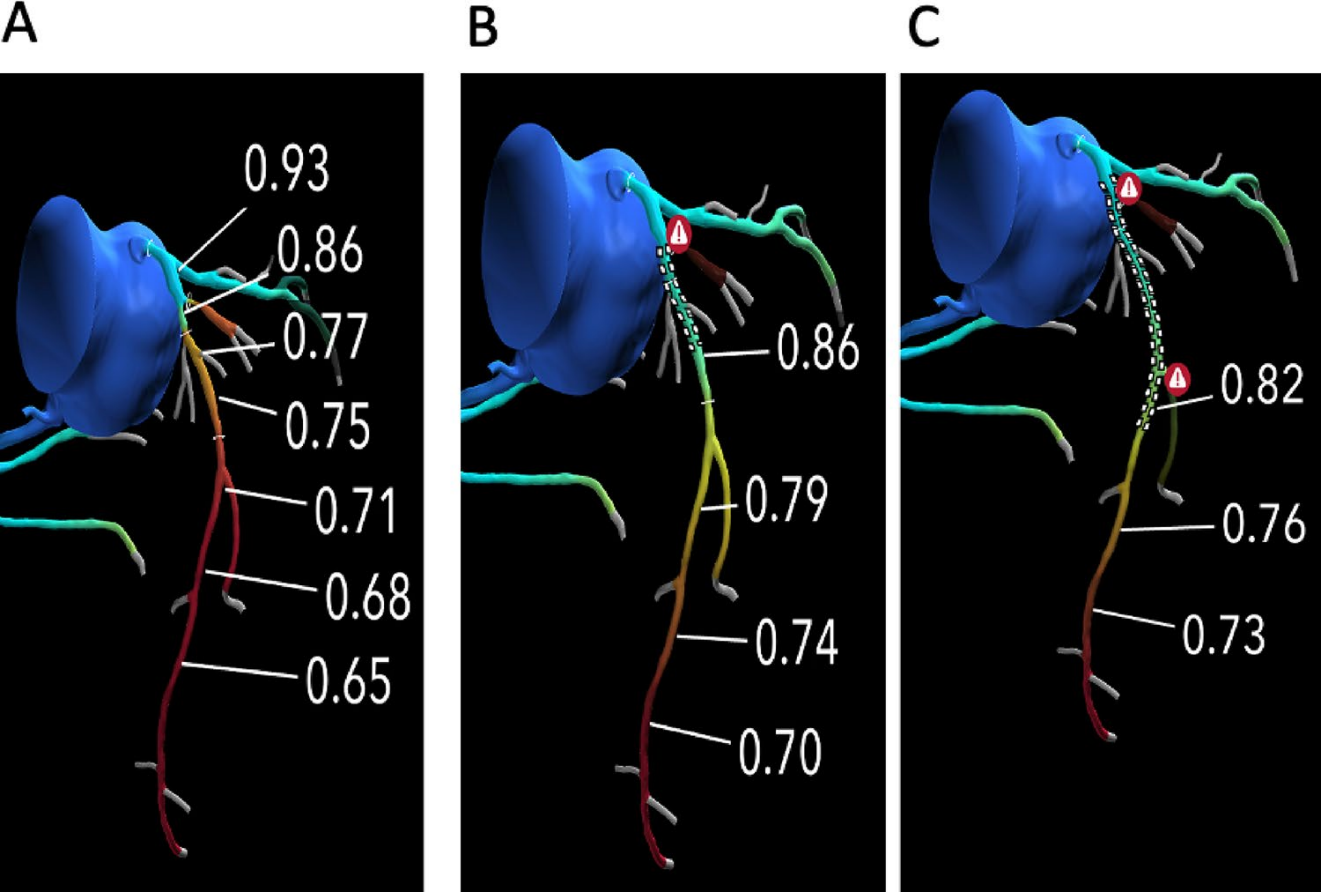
(A) CTCA with an anatomical severe stenosis and subsequent moderate stenosis in the LAD. (B) Modelled PCI with post PCI FFR_CT_ improving to 0.86 across the proximal lesion but FFR_CT_ <0.8 distally. (C) Modelled extension of the stented segment across both lesions with a post PCI-FFR_CT_ remaining sub-optimal. Indicative of diffuse coronary artery disease unlikely to benefit from PCI. Abbreviations: CTCA = computed tomography coronary angiogram.FFR_CT_=CT based fractional flow reserve.PCI = percutaneous coronary intervention. LAD = left anterior descending
